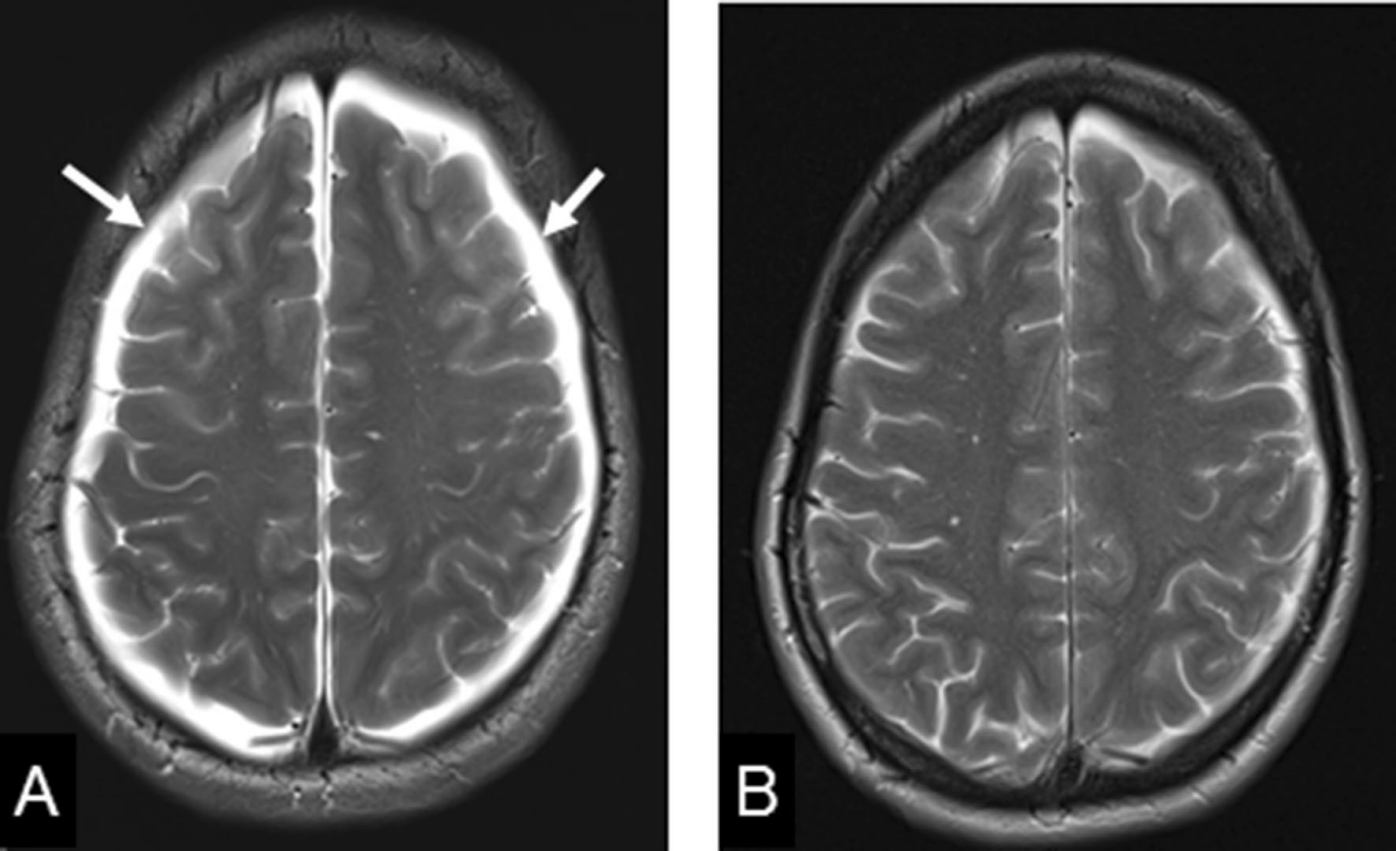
a Bilateral subdural hygromas in T2 weighted MRI (arrow); b significantly improved hygromas according to postoperative MRI
